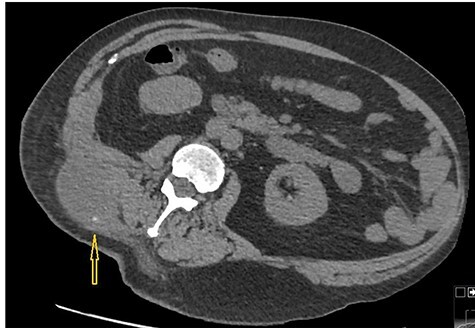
Non-contrast CT abdomen: CT scan demonstrates a small hyper dense calculus in the abscess marked by an arrow. Here patient is lying in slightly left lateral position due to pain caused by the abscess.
